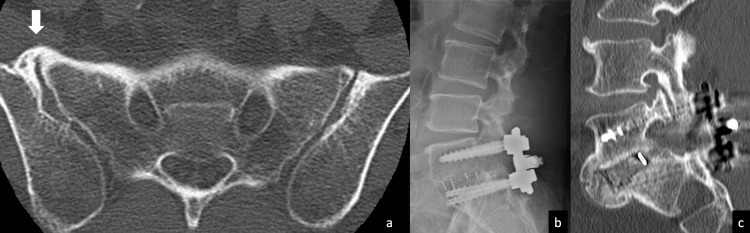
Case 2: A case with anterior bony ankylosis of the right sacroiliac joint. Bone fusion was achieved one year after surgery: (a) preoperative axial CT slice showing bony ankylosis of the right sacroiliac joint. The white arrow indicates the bony bridge. (b) Postoperative X-ray during extension showing no sign of bone fusion failure around the endplates. (c) Postoperative sagittal CT showing a bone bridge between the L5 and S1 endplates.
